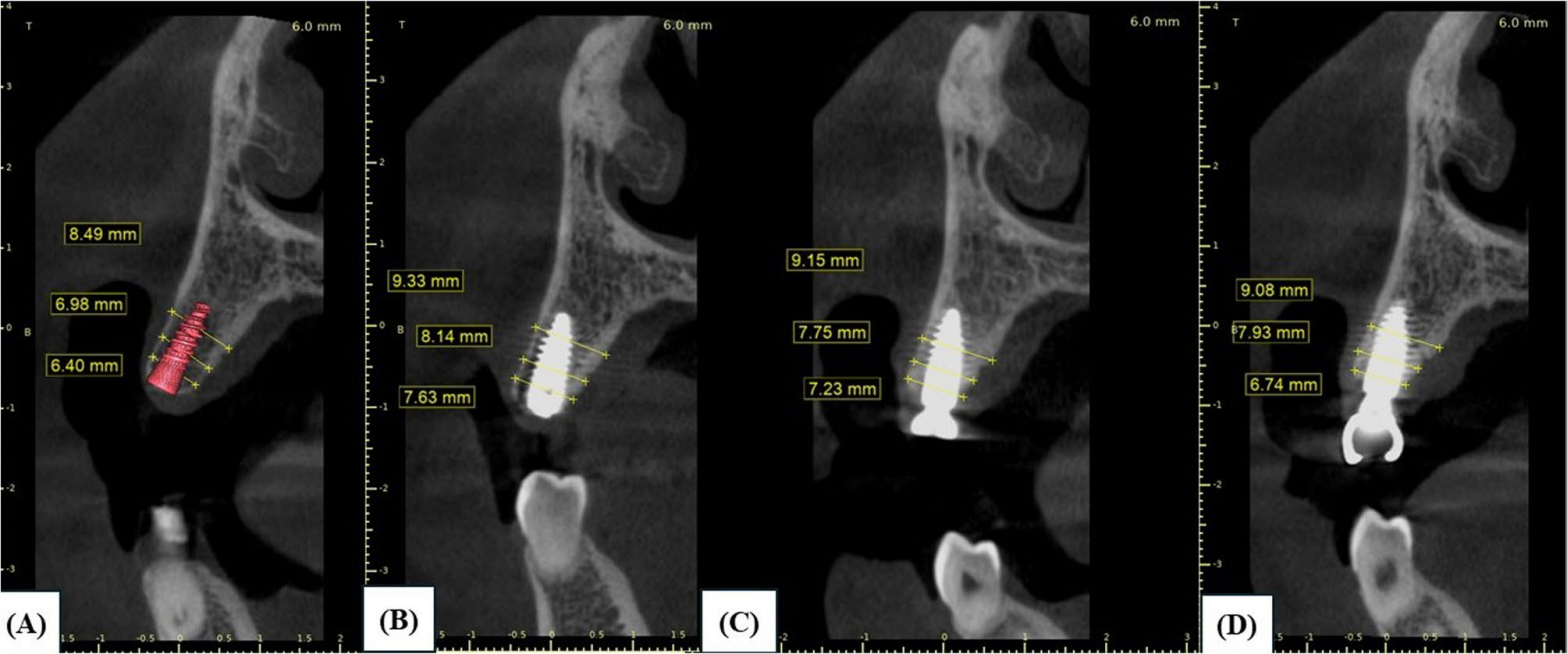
Radiographic assessment of the ridge width at different vertical levels A-D. A Before implant placement. B Baseline (immediately post-operative).C 3-month follow-up. D 6-month follow-up
